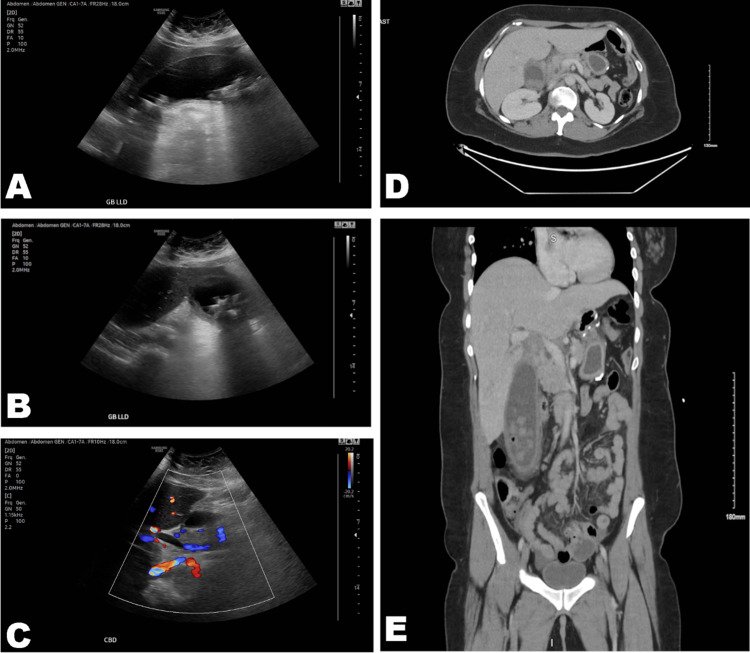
Ultrasound and computed tomography scans. (A, B) An oblique sonogram shows an enlarged gallbladder with several gallstones. (C) A color Doppler sonogram shows a common bile duct of 8 mm. (D, E) Computed tomography scans show an enlarged gallbladder with several gallstones and hepatomegaly.
